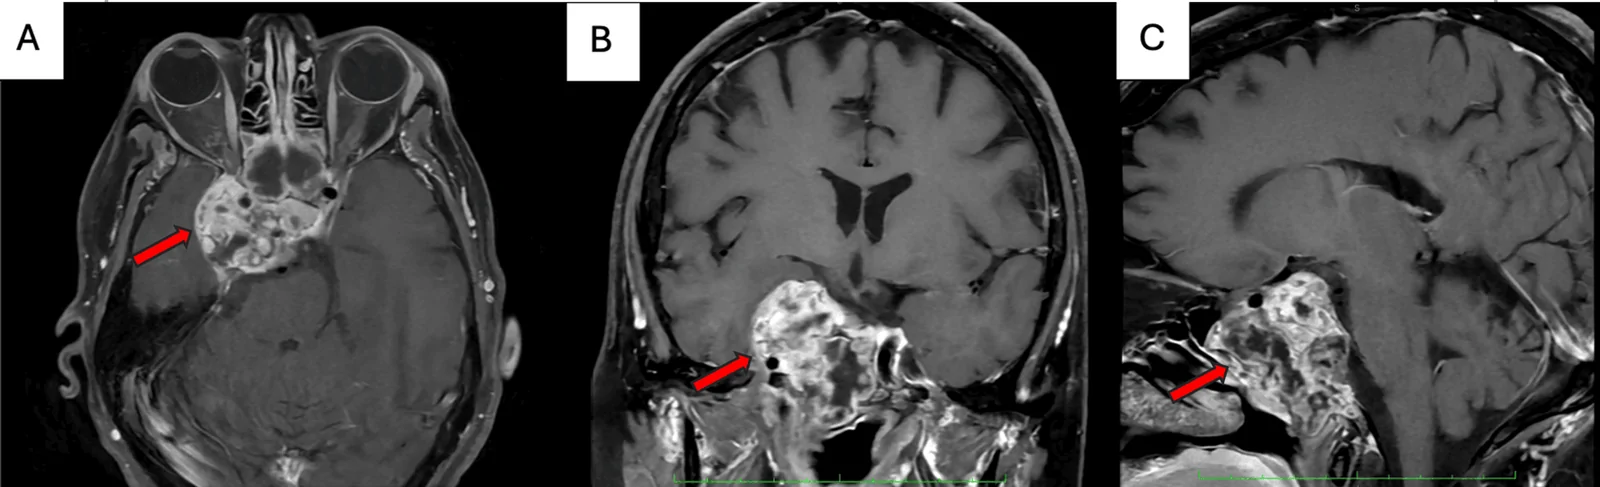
Follow-up MRI demonstrating lesion progression in August 2025 (A) Axial, (B) coronal, and (C) sagittal contrast-enhanced T1-weighted MR images obtained in August 2025 demonstrate a large, heterogeneous, expansile lesion centered in the clivus, with extension into the sphenoid sinus and right cavernous sinus. The lesion measured 5.7×5.1×4.8 cm, compared with 5.6×4.8×4.3 cm on MRI obtained in May 2025. Red arrows indicate the lesion in each plane.
